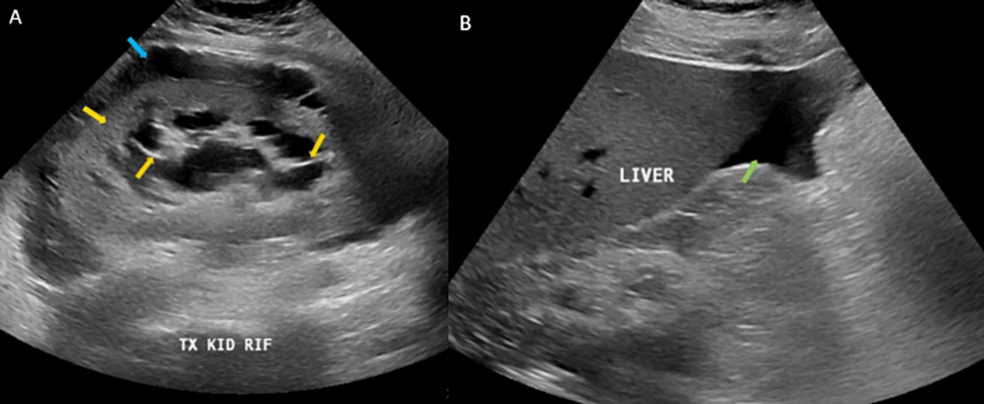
Gray-scale ultrasound-selected images of the transplanted kidney and upper abdomen show an increased parenchymal echogenicity of the kidney (yellow arrow), with renal peri pelvicalyceal cystic structures and hyperechoic wall/septae (orange arrows). There is a perinephric fluid collection with septae (blue arrow) along with minimal subhepatic free fluid (green arrow).
